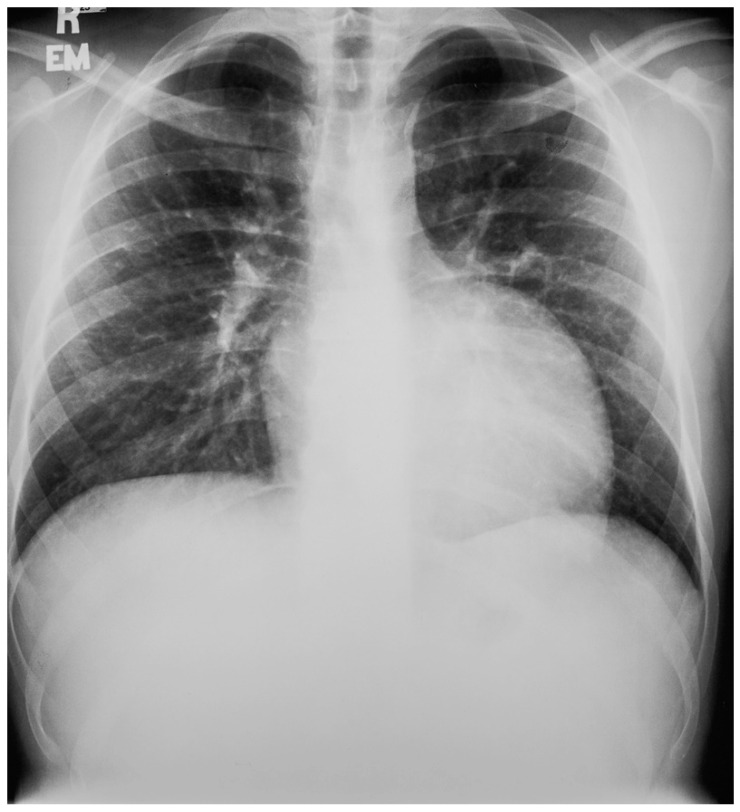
Posteroanterior chest X-ray in congenitally corrected transposition of the great arteries: This 20-year-old male presented with dyspnea on exertion. The image is well centered and there is a good inspiration. There is an increased cardiothoracic ratio, due to a pronounced left heart border attributed to an enlarged systemic ventricle. There is a double shadow on the right heart border due to left atrial enlargement. There is a narrow waist between the great arteries and the heart that is made more evident by the enlarged cardiac silhouette. By palpation, the cardiac apex was laterally displaced. There was a grade 3/6 holosystolic murmur of systemic atrioventricular regurgitation at the cardiac apex. Doppler echocardiography demonstrated a markedly dilated systemic ventricle, with severe systemic atrioventricular regurgitation into a dilated left atrium consistent with congenitally corrected transposition of the great arteries; no other congenital cardiac anomaly was identified. Medical treatment for heart failure was initiated.
